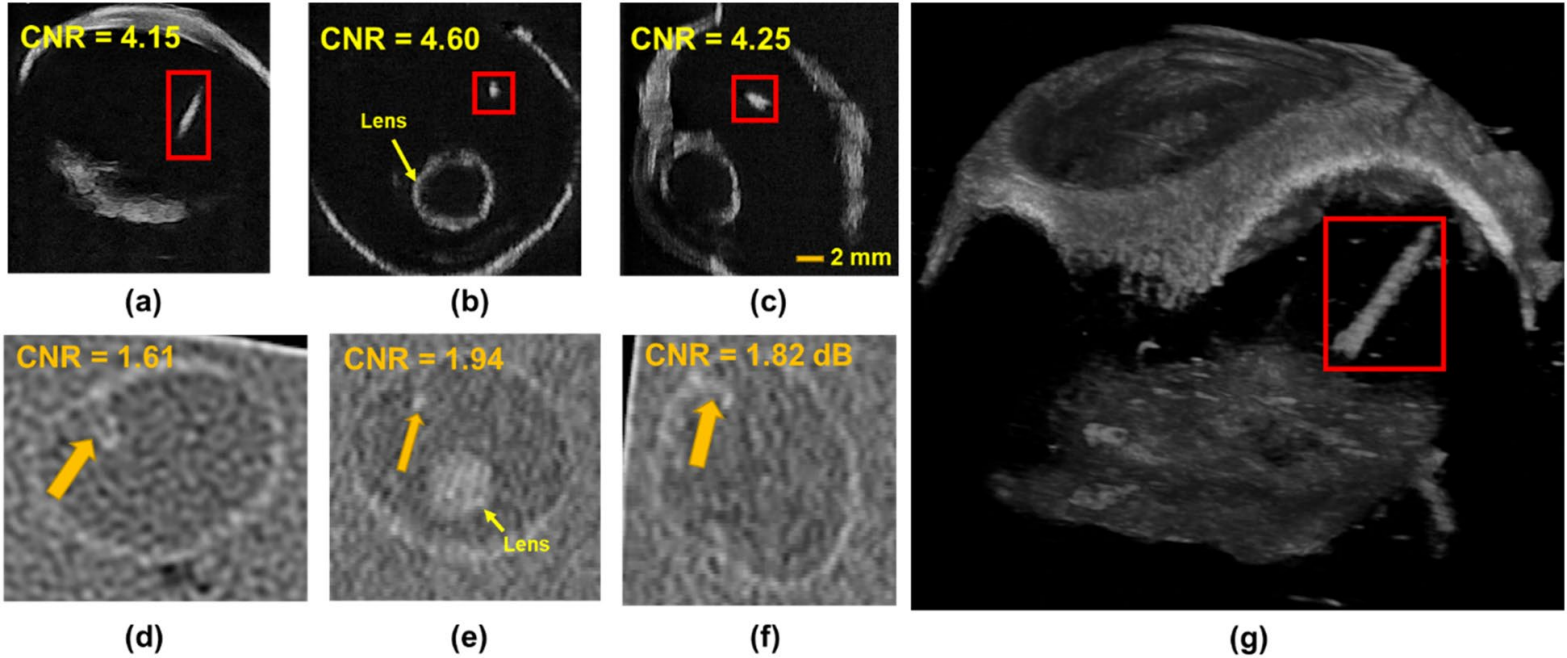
Visualization of wooden IOFB in 3D-UBS and CT. (a)–(c) and (d)–(f) show 2D views of the same wooden IOFB using 3D-UBS (red box) and CT (yellow arrow), respectively. Due to vitreous being anechoic to ultrasound, 3D-UBS shows superior contrast compared to CT in all three planes. (g) shows 3D visualization of the entire wooden IOFB.
